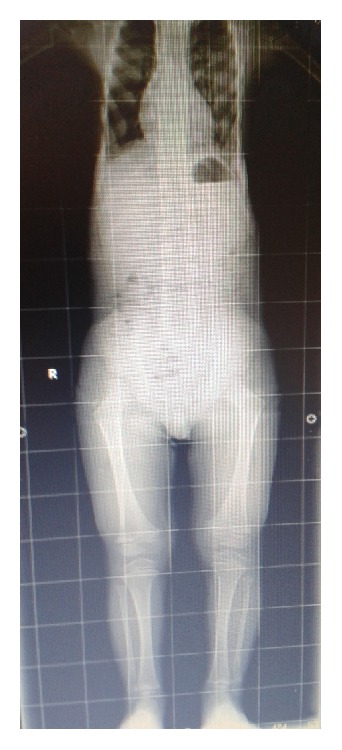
Radiography of patient. A long and narrow thorax, short and horizontal costae, and short extremities.
